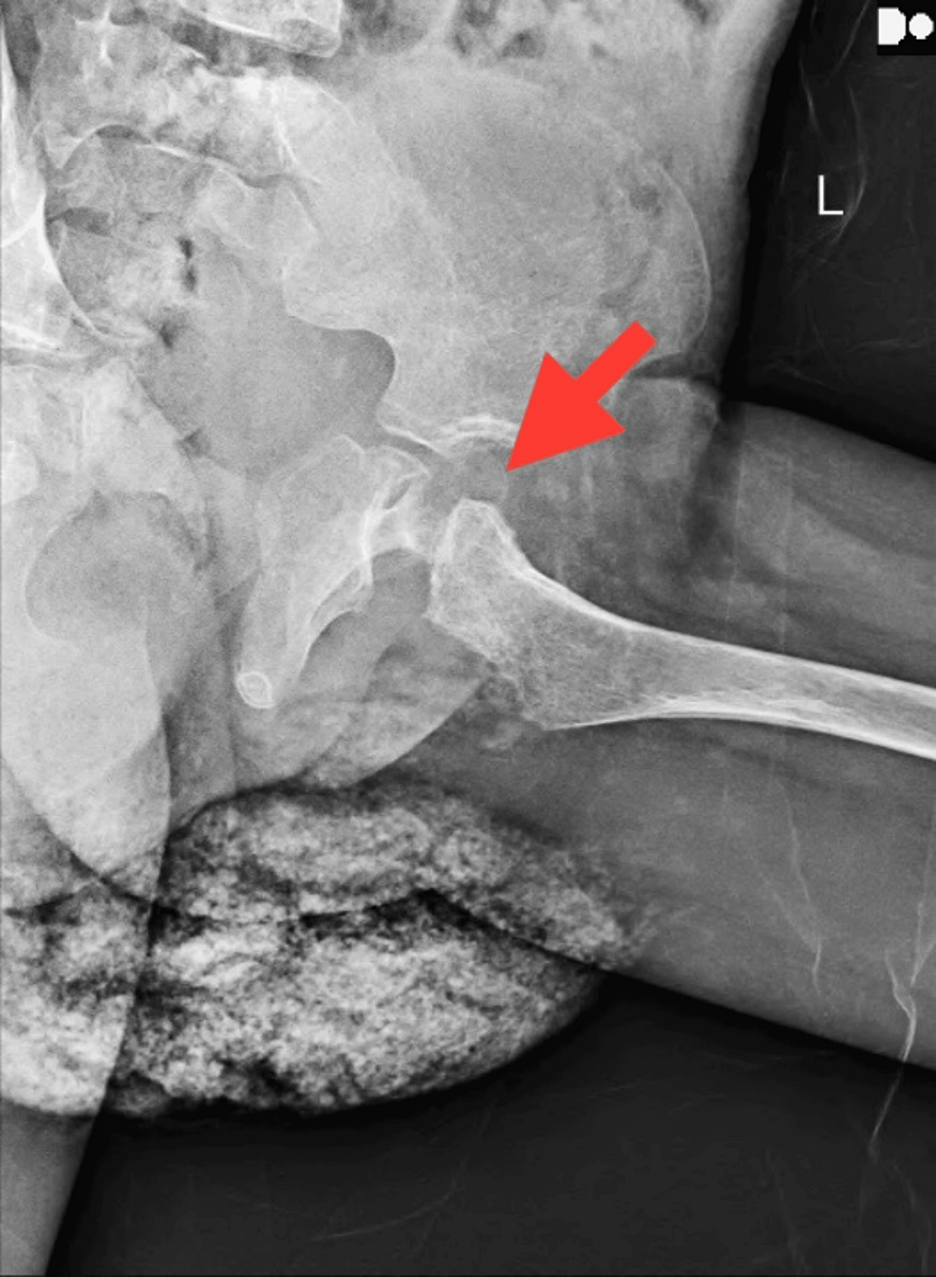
Radiographic imaging of left hip joint
